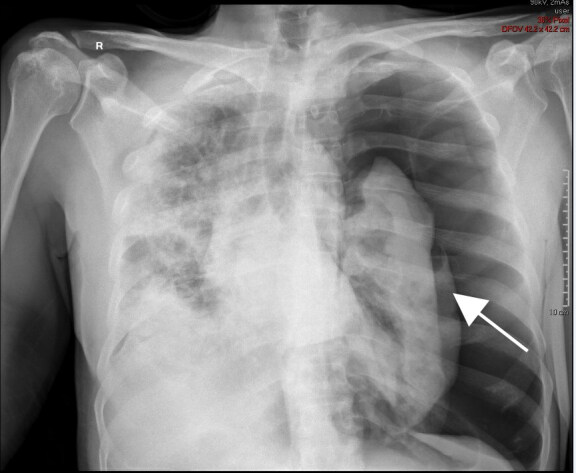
Tension pneumothorax of the left lung on chest radiograph. Arrow indicates compressed left lung surrounded by free air.
